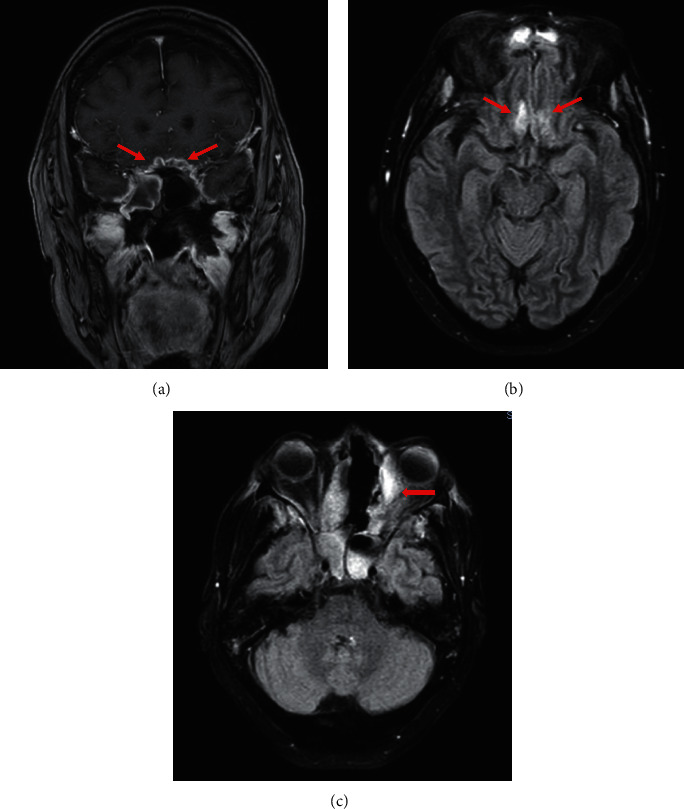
(a) Coronal T1-weighted contrast-enhanced MRI reported frontal anterobasal dural thickening and strong pachymeningeal enhancement (arrows). (b) Fluid attenuated inversion recovery (FLAIR) sequence showed hyperintense signal in cortical anterobasal frontal lobes (arrows). (c) FLAIR sequence revealed an abscess adjacent to the inferior rectus muscle of the left orbit (red arrow).
